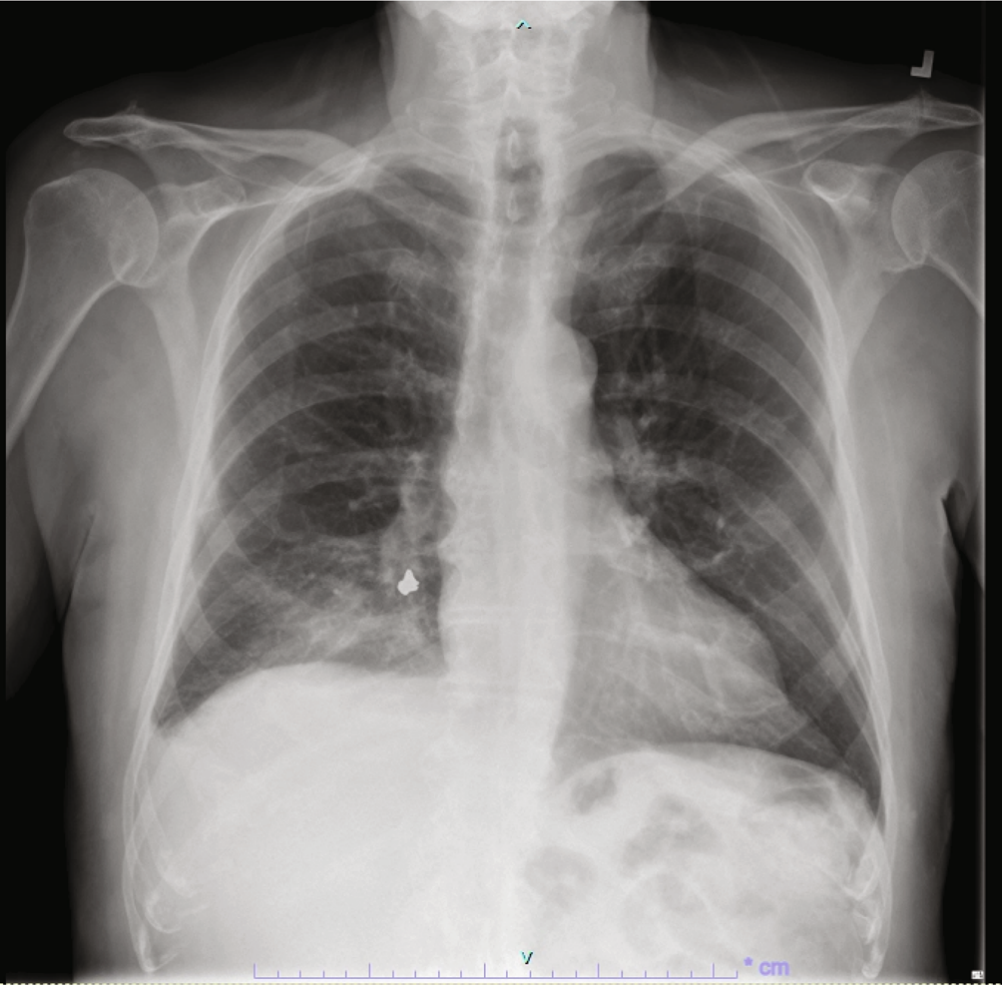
Anterior/posterior chest X-ray demonstrating radiopaque foreign body.
